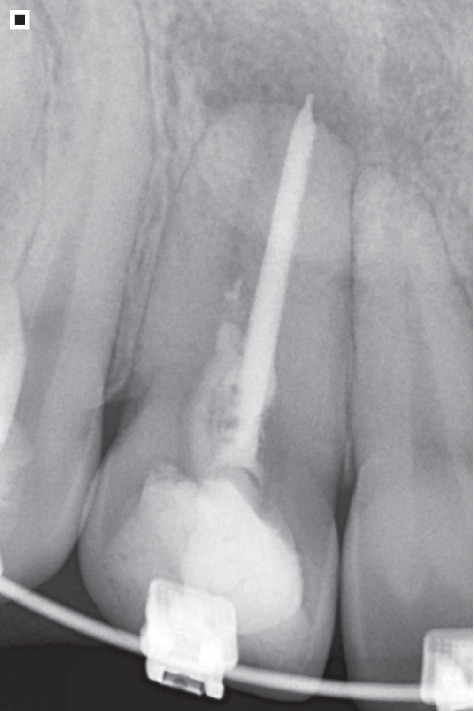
Periapical radiograph of Tooth 12.
